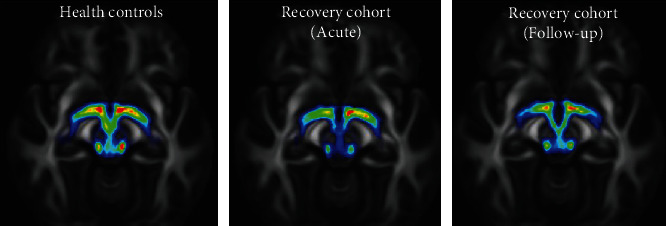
Brainstem MRI from OpenNeuro dataset.
